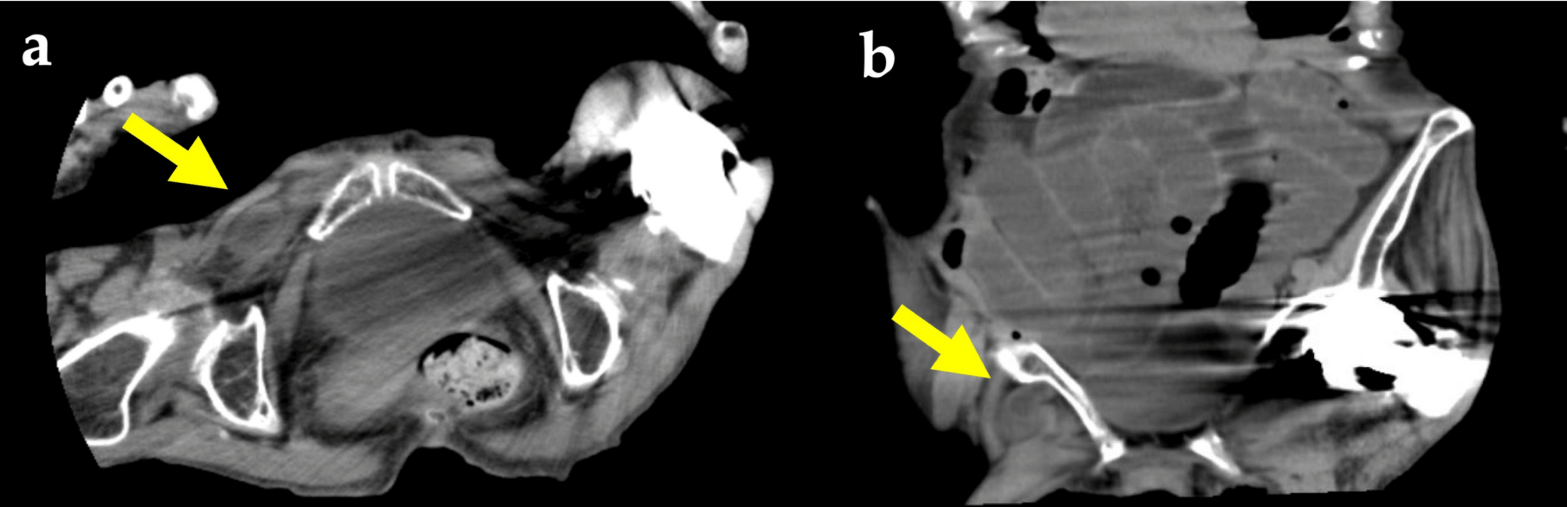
Abdominal computed tomography scan showing right obturator hernia incarceration (arrows): (a) axial view; (b) coronal view.
